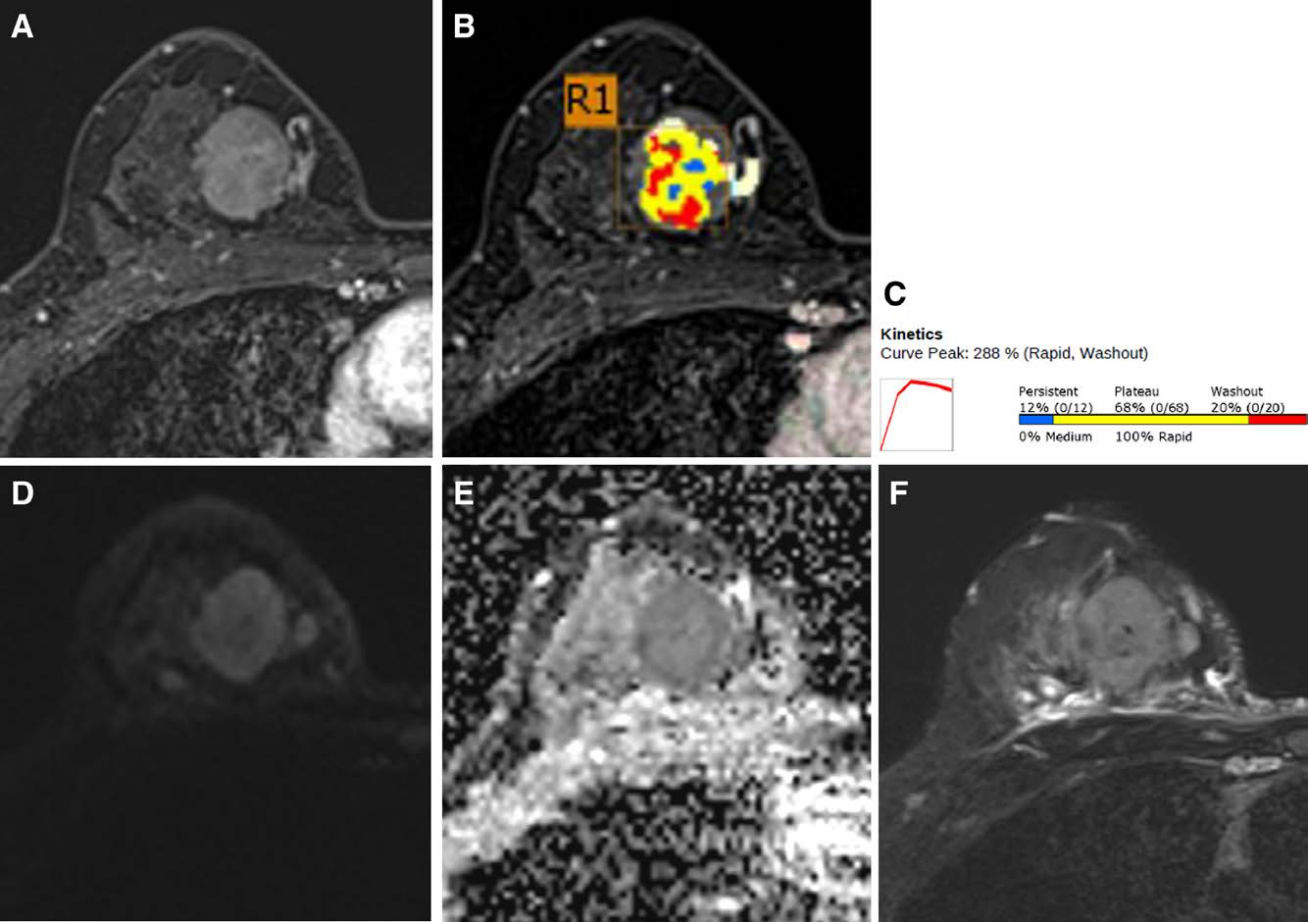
(A) Preoperative axial fat-suppressed T1-weighted contrast-enhanced MR image showing a 3.4 × 2.7 × 3.0 cm irregular, heterogeneously enhancing mass in the upper inner quadrant of the right breast. (B) MR image with CAD color overlay map demonstrating tumor enhancement kinetics. Red, yellow, and blue areas indicate washout, plateau, and persistent enhancement patterns, respectively. (C) Kinetic curve graph showing rapid initial enhancement and rapid washout-type curve. The initial peak enhancement value is 288%. In the delayed phase, 20% of the mass shows washout, 68% of the mass shows a plateau-type, and 12% shows a persistent-type enhancement. (D) T2-weighted image showing no cystic component in the mass. (E and F) Diffusion-weighted image (*b* = 1000) and ADC map show no significant diffusion restriction. ADC = apparent diffusion coefficient; CAD = computer-aided detection, MR = magnetic resonance.
